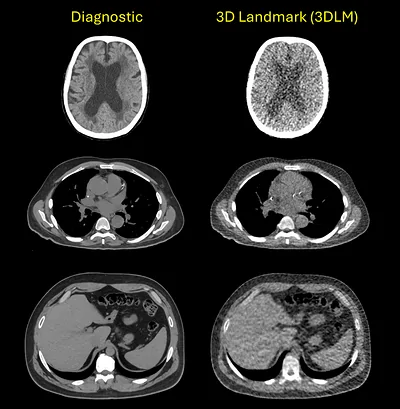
Example central axial slices through diagnostic non‐contrast (NC) CT and 3D landmark (3DLM) scans for Head, Chest, and Abdomen‐pelvis body regions. All images are shown with the same soft tissue window/level setting (W/L = 400/40).
